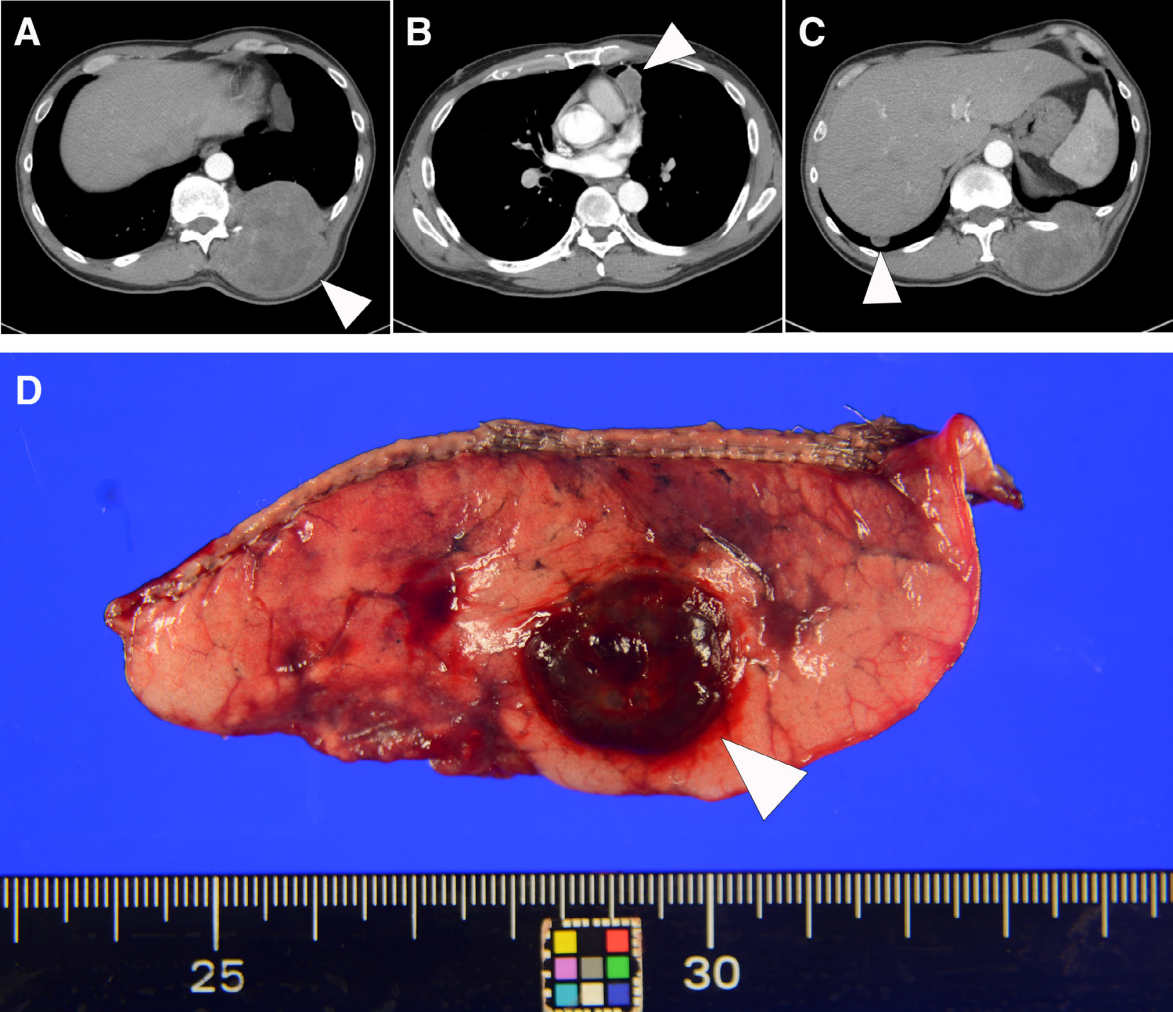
(A–C) Contrast‐enhanced computed tomography. (A) Osteolytic tumor with a diameter of 73 mm on the left 11th rib (arrowhead). (B) Tumor in the mediastinum (arrowhead). (C) Tumor in the lung (arrowhead). (D) Resected lung displaying a dark reddish‐brown hemorrhagic nodule (arrowhead).
